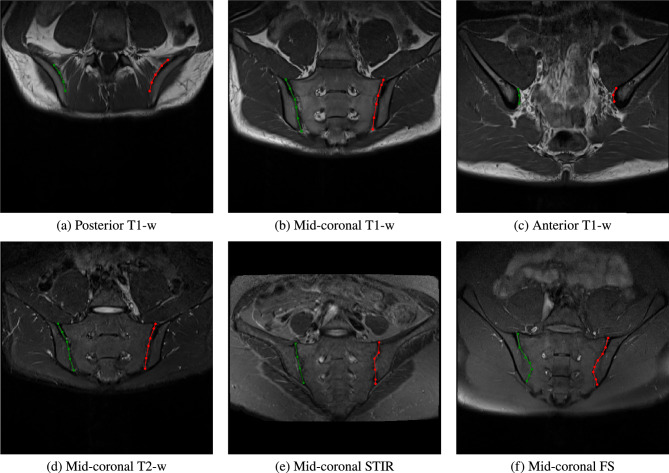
Example scans in the dataset with their marked-up annotated landmarks. (a–c) Slices from the same T1-weighted scan at differing slice positions (anterior, mid-coronal, posterior) while (d–f) are mid-coronal examples of different sequences in the dataset.
